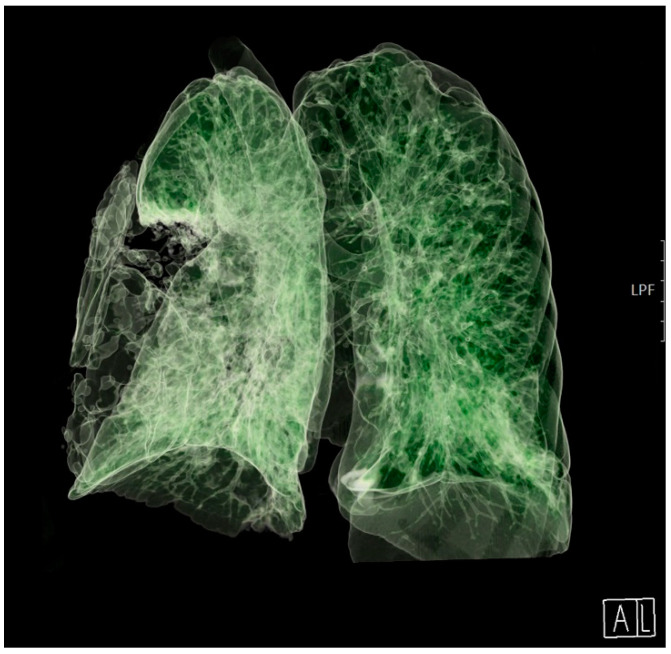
A 3D computed tomography scan of the lungs 25 days after admission to the Intensive Care Unit. A: anterior, L: lateral.
